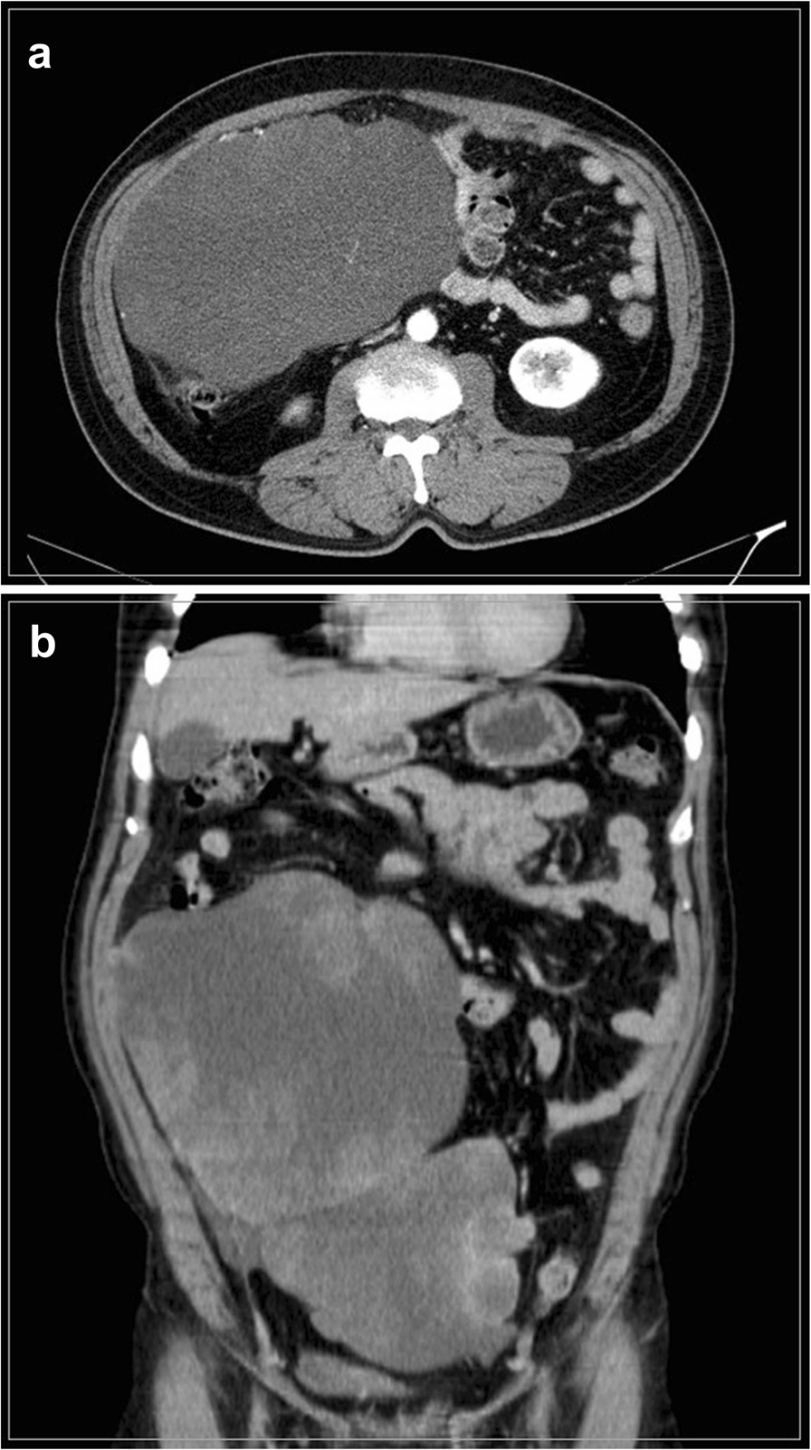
Computed tomography scan showing the presence of a huge mass lesion in the right mid to lower abdomen extending to the pelvic cavity. a Coronal view. b Sagittal view
